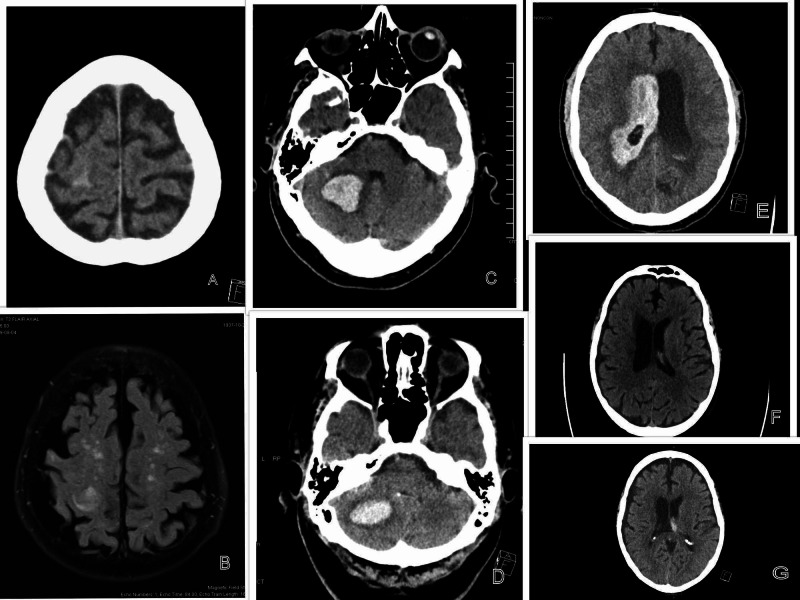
Image showing intracranial hemorrhage in patients at presentation and 12 hours after receiving andexanet (Case 1) A: CT scan at presentation with a small subarachnoid hemorrhage in the right frontoparietal lobe, B: MRI 12 hours later, showing the unchanged subarachnoid hemorrhage in the right frontoparietal region. (Case 2) C: CT scan showing a cerebellar hemorrhage at presentation, D: CT scan at 12 hours with the cerebellar hemorrhage reduced in size. (Case 3) E: CT scan of the head at presentation showing a diffuse intraventricular hemorrhage. (Case 4) F: CT Scan at presentation showing a small amount of hemorrhage in the left intraventricular foramen, G: CT scan at 12 hours showing increased intraventricular hemorrhage in the left intraventricular foramen. CT: computed tomography; MRI: magnetic resonance imaging
